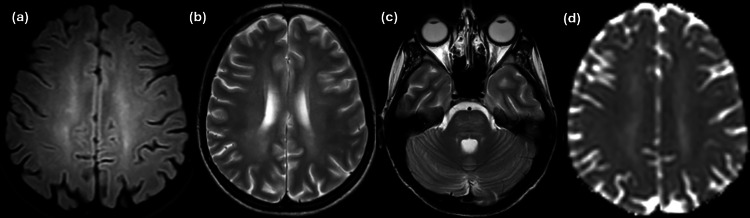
(A). Axial FLAIR image showing diffuse high signal intensity involving white matter of the centrum semiovale. U-Fibers are spared. (B, C). Axial T2 weighted image showing diffuse, almost symmetric high signal intensity involving periventricular and deep white matter (B) and pons (C). (D). Apparent Diffusion Coefficient (ADC) maps reveal the involved periventricular and deep white matter areas with increased diffusivity.
